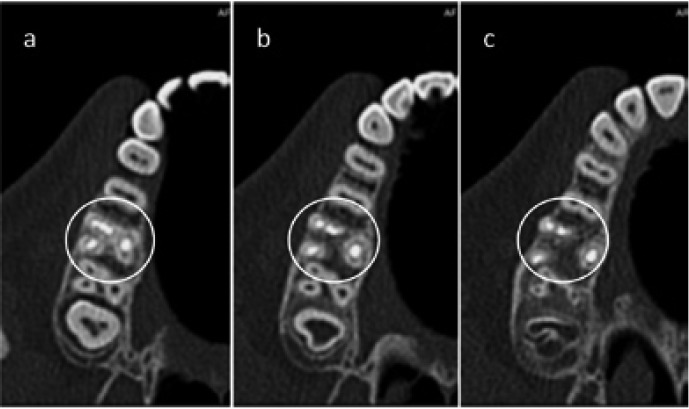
Spiral CT images; a.Cervical third; b.Middle third; c.apical third.
